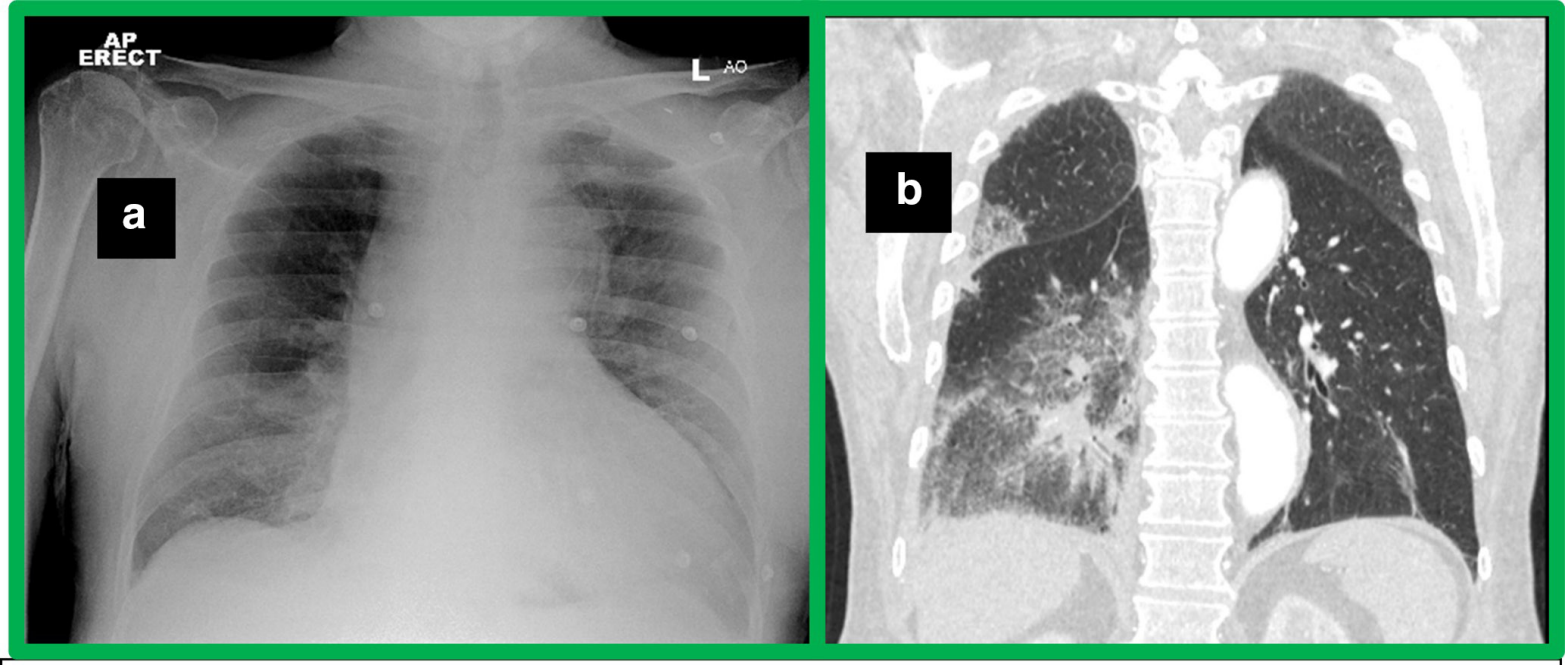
a. AP chest radiograph demonstrates airspace opacification in the right lung
